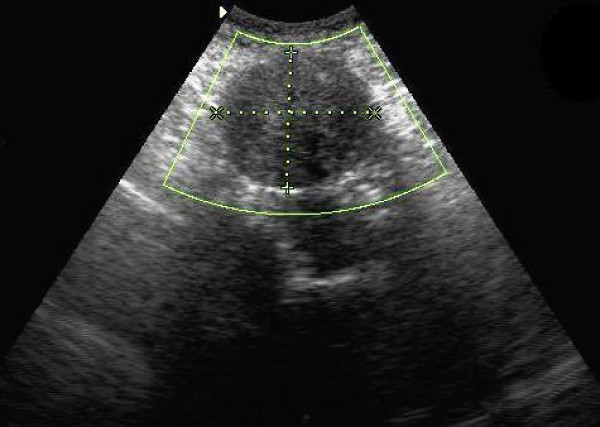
Ultrasound of the abdomen, left lower quadrant. US shows a large, rounded, well-defined hypoechoic mass in the left lower quadrant abdomen.
